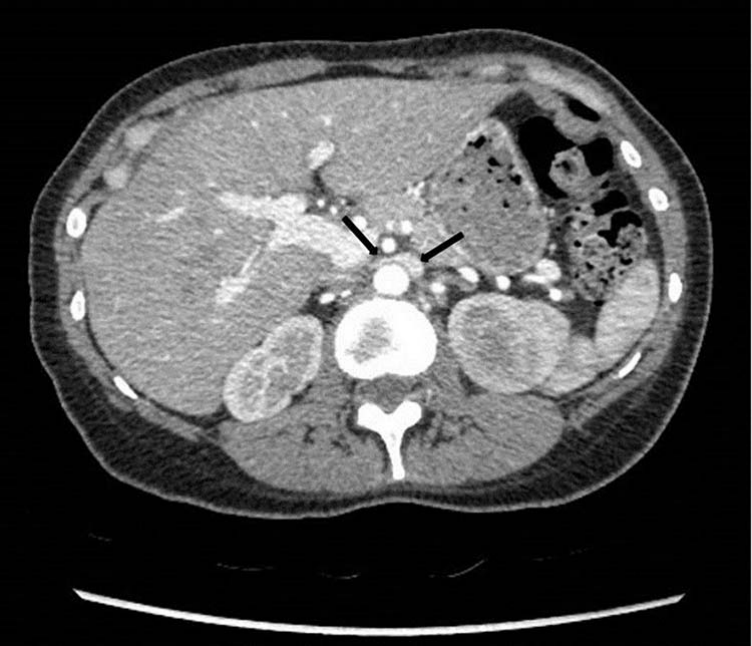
Computed tomography angiogram on presentation showing pre-compressed LRV (right arrow); and compressed LRV (left arrow).
